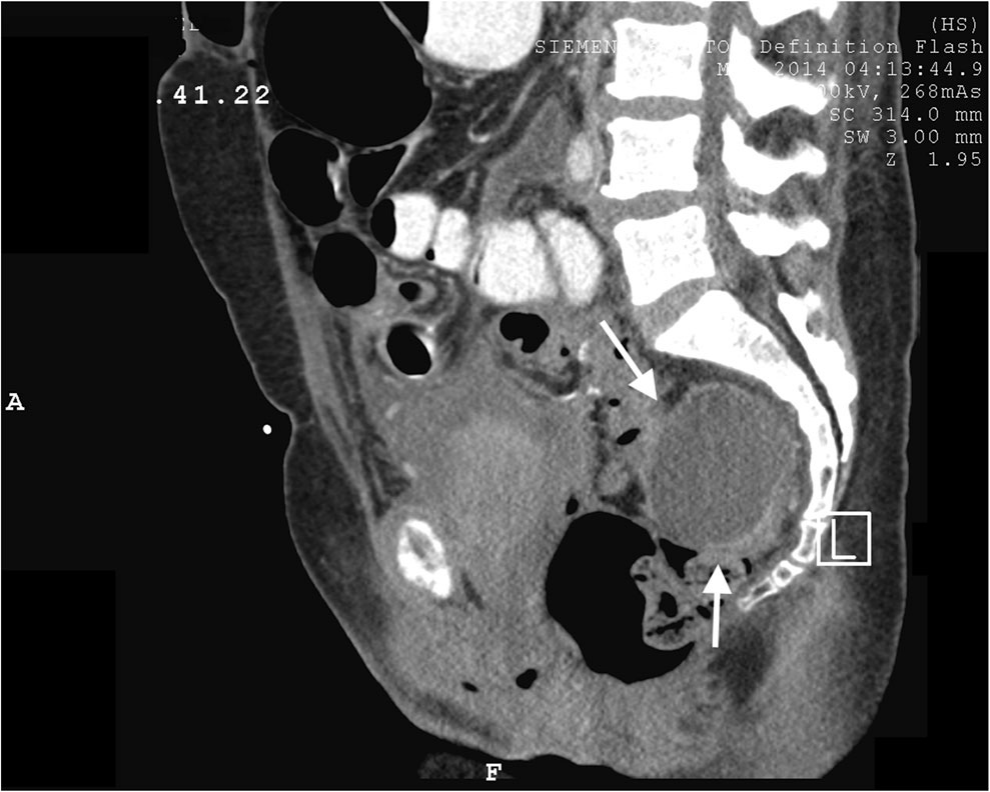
From patient #3. A sagittal midline image from the initial oral and intravenous contrast-enhanced CT. This demonstrates the rounded posterior pelvic fluid collection, 8.5 × 5.6 × 2.6 × 2.3 cm, with minimal marginal enhancement, consistent with an abscess (*arrows*)
